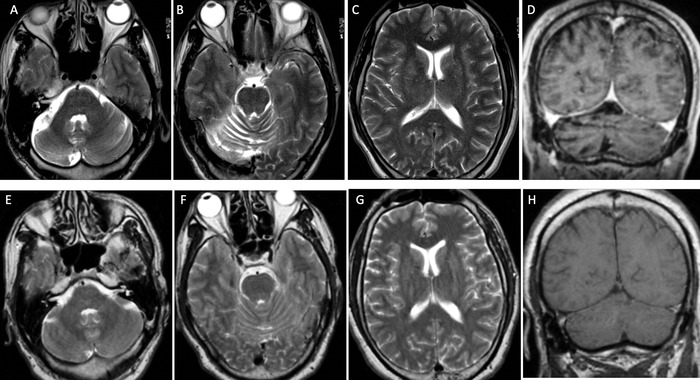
Magnetic resonance imaging of the brain from 2021 includes (A-C) axial T2 and (D) coronal contrast-enhanced T1 images showing diffuse volume loss throughout the right cerebellar hemisphere with expansion of cerebrospinal fluid spaces and widening of cerebellar sulci (A, B, D) that were greater than expected for the patient's age. (C) Supratentorial brain volumes are normal. Corresponding comparison images from 2011 (E-H) are normal with no cerebellar atrophy.
